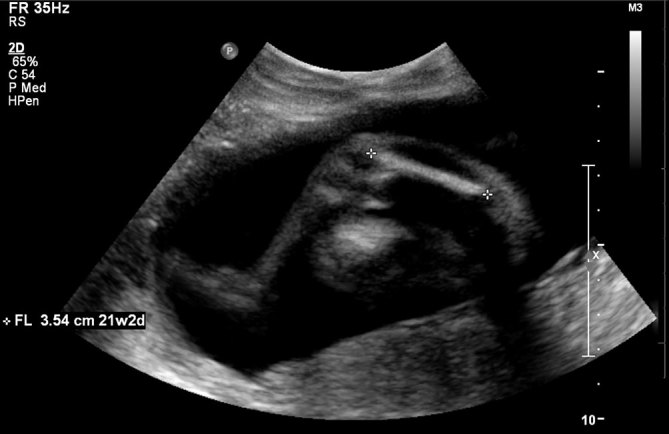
Prenatal ultrasound at 22 6/7 weeks gestational age demonstrates short femurs measuring 3.5 cm (< 2.5%).
